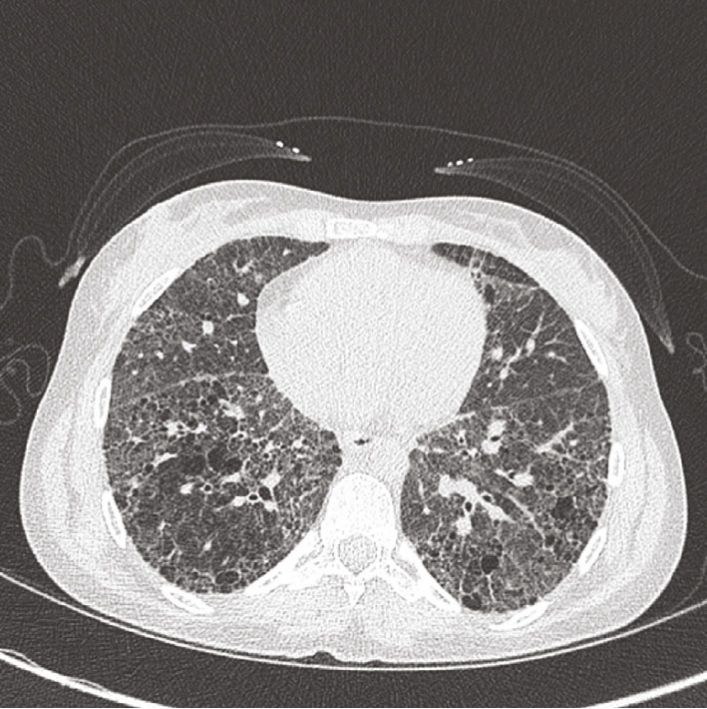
Chest computed tomography of the patient's native lungs demonstrating cystic changes, diffuse coarse reticular interstitial thickening with associated ground glass opacities.
